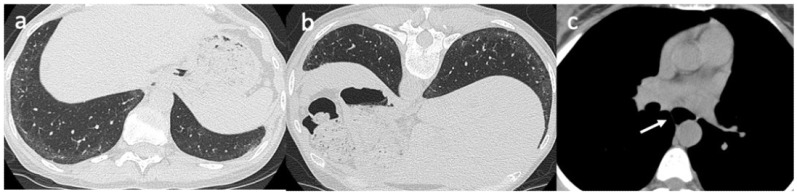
A 43-year-old female with a diagnosis of systemic sclerosis. Axial high-resolution CT scan obtained in the supine position shows subtle ground-glass opacities in the subpleural regions of the lung bases, suspicious for NSIP (non-specific interstitial pneumonia) (a). When these findings are not prominent, an additional scan can be acquired in the prone position to differentiate ground-glass opacities due to gravitational phenomena from interstitial lung disease. In this patient the ground-glass opacities persist in the prone position, confirming the interstitial lung involvement (b). Additional systemic sclerosis-related findings should be searched for, such as a dilated esophagus on images reconstructed using an appropriate mediastinal window setting (c).
